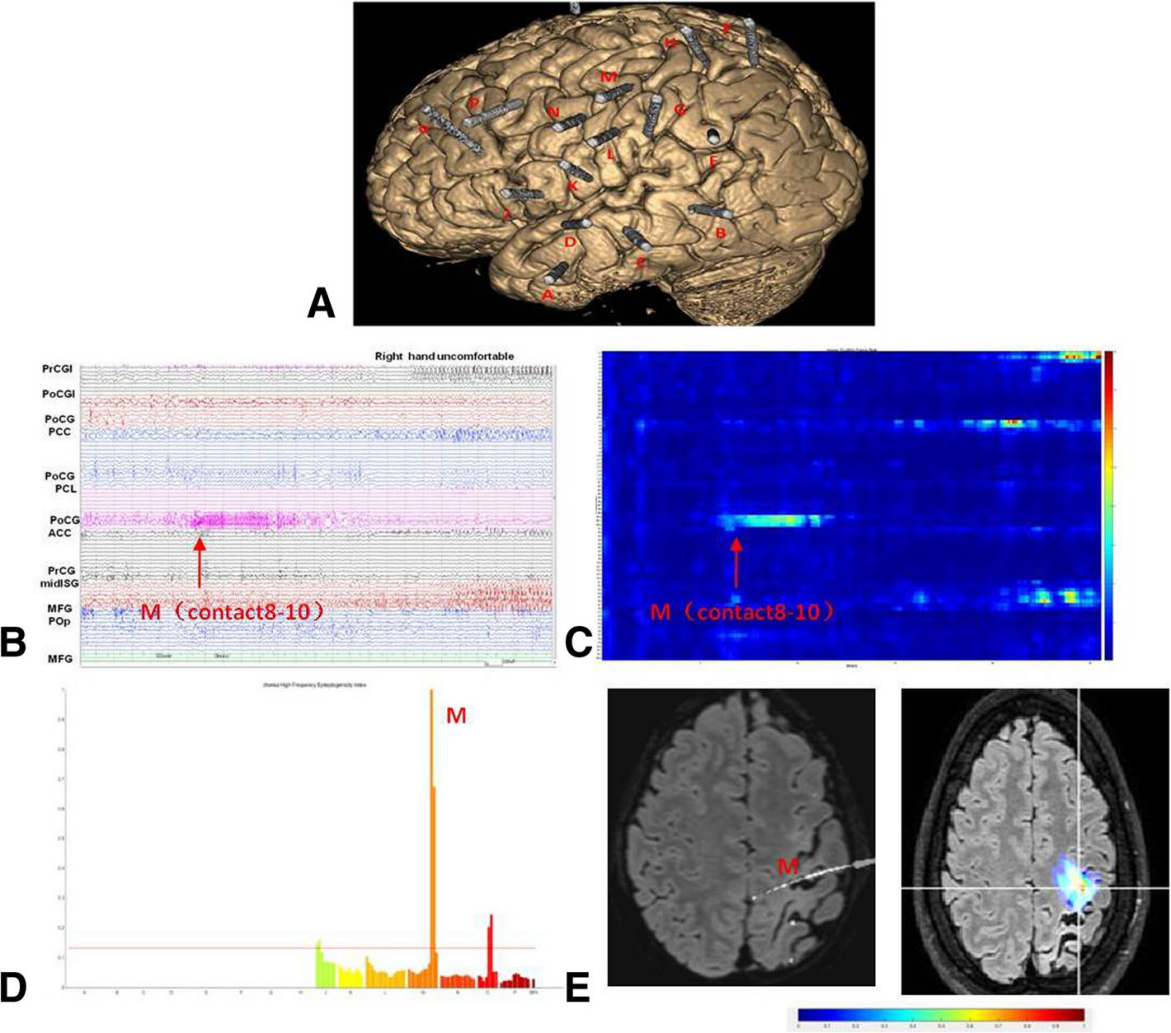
SEEG recordings of Patient 3. a Location of the SEEG electrodes shown on the 3D MRI image, The electrodes mainy explored perisylvian region of left hemisphere. b Seizures started with low voltage fast activities in the postcentral gyrus (external leads of electrode M). c Axis X indicates time of selected seizure, axis y indicates electrodes, ER(Energy Ratio)of high frequencies changes was depicted in a color scale (From red to blue ER intensity decreased), Quantification of the seizure onset was in the same position(electrode M) as visually seen. d Axis X indicates electrodesrodes, axis Y indicates EI values, highest EI quoted on the chart demonstrating maximal epileptogenicity in the electrode M. e EI values were superimposed on the axial planes of MRI, (color scale:From red to blue EI value decreased),seizure onset was confined to a single gyrus. PrCGI:precentral gyrus of insular; PoCGI:postcental gyrus of insular; PCC:posterior cingular cortex; PCL:paracentral lobule; PoCG:postcentral gyrus; PrCG:precentral gyrus; Pop:parietal operculum MFG:middle frontal gyrus
